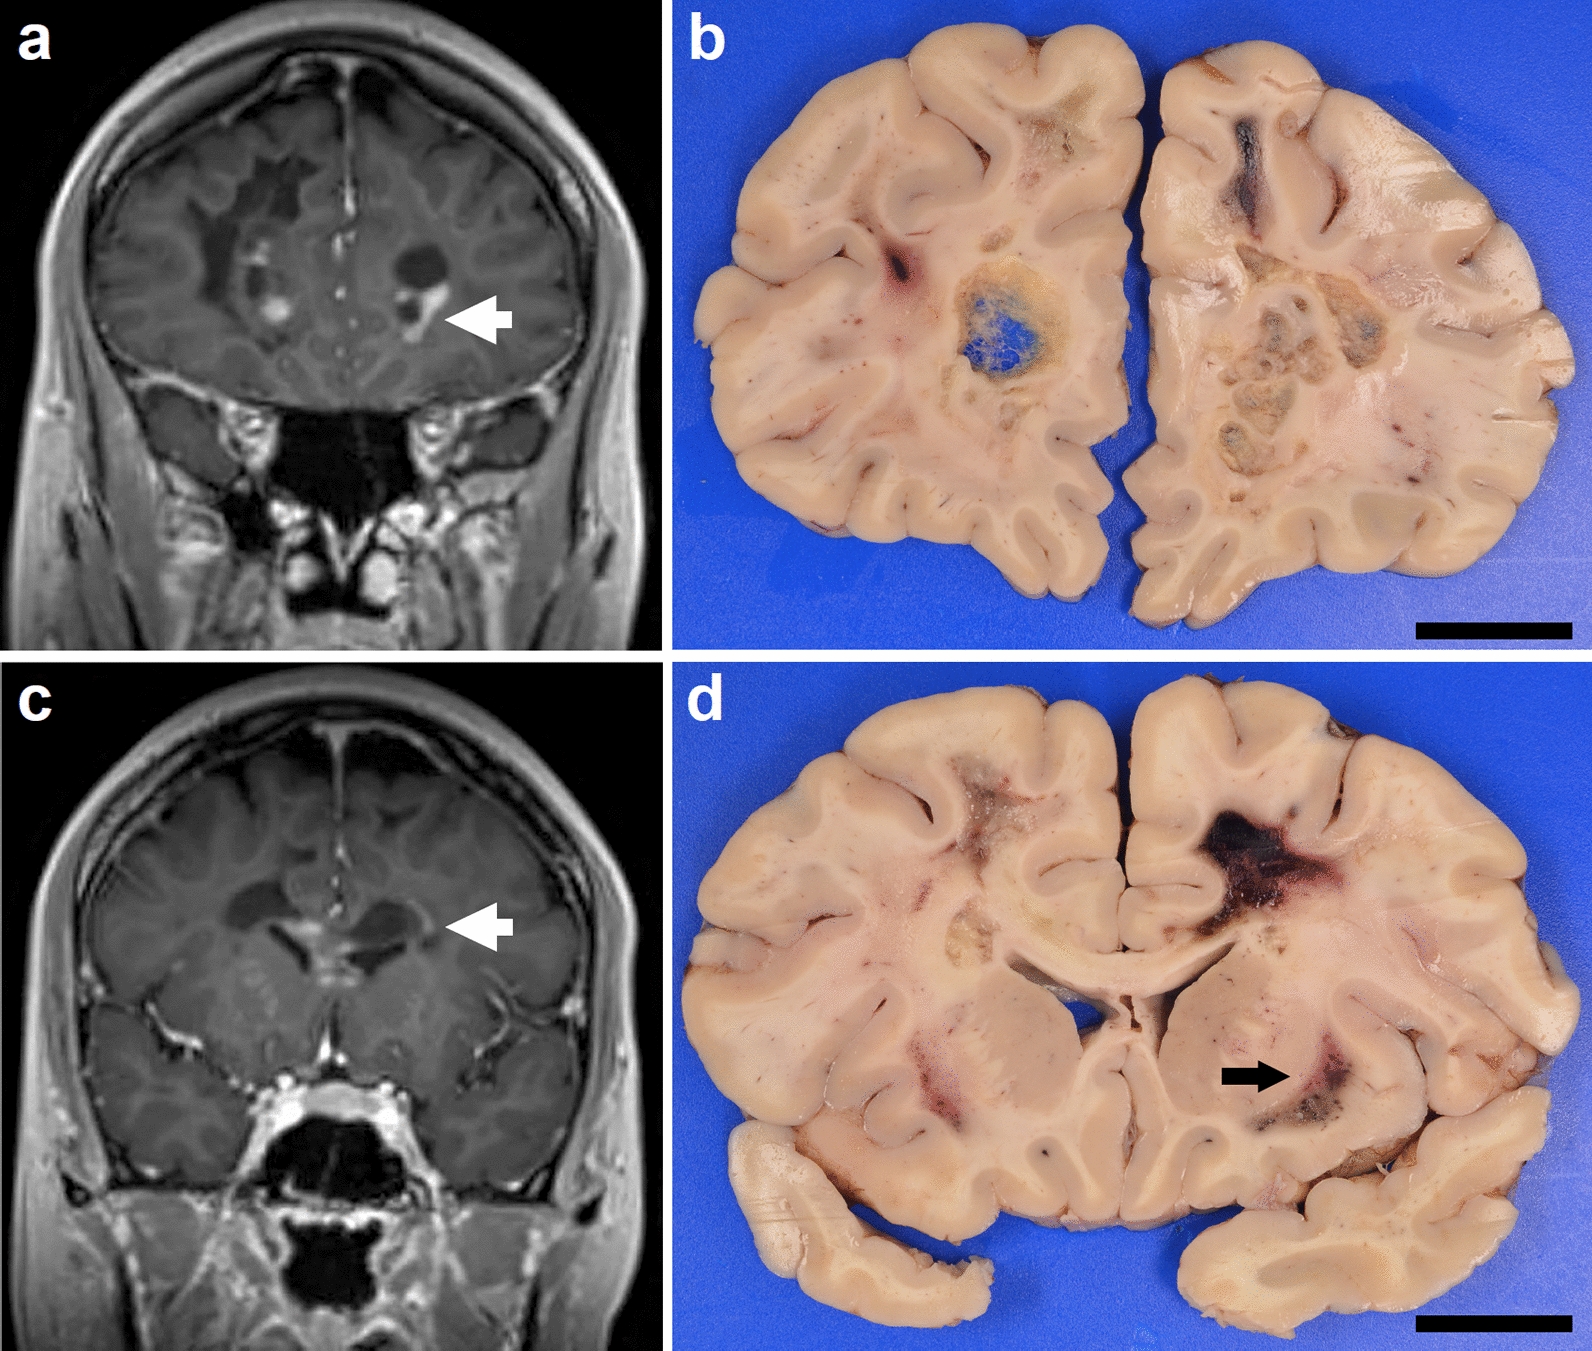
Radiologic and pathologic findings at autopsy. MRI performed several weeks before death showed cystic changes (white arrow) in the periventricular white matter with associated areas of post-contrast enhancement a, c. Gross examination at autopsy showed numerous cystic lesions in the periventricular white matter b, as well as solid lesions characterized by softening and dusky discoloration (black arrow) d. Size bar in panels b and d are 2 cm
